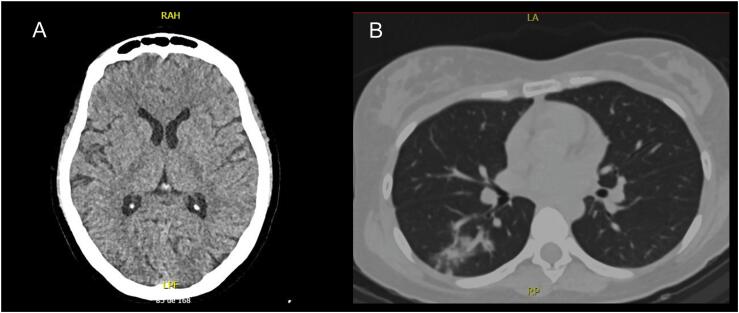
Computed tomographic images of the patient: (A) brain scan and (B) lung scan.
